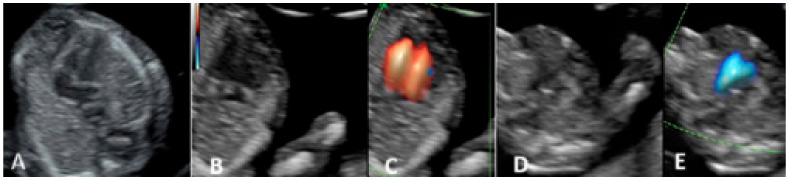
First-trimester heart protocol. (A) Four-chamber view. (B) Atrio-ventricular flows—Grey Scale. (C) Atrio-ventricular flows—Color Doppler Mode. (D) 3-vessel view—Grey Scale. (E) 3-vessel view—Color Doppler Mode.
